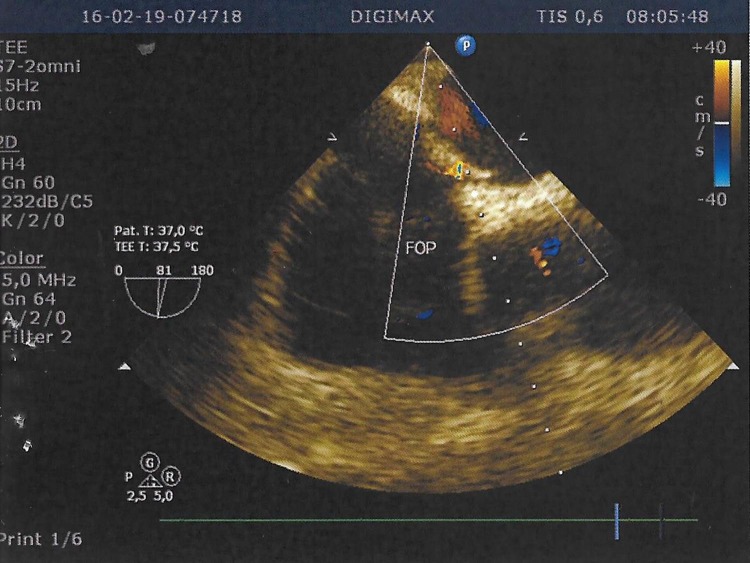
Transesophageal bubble study echocardiogram showing a patent foramen ovale (FOP).
